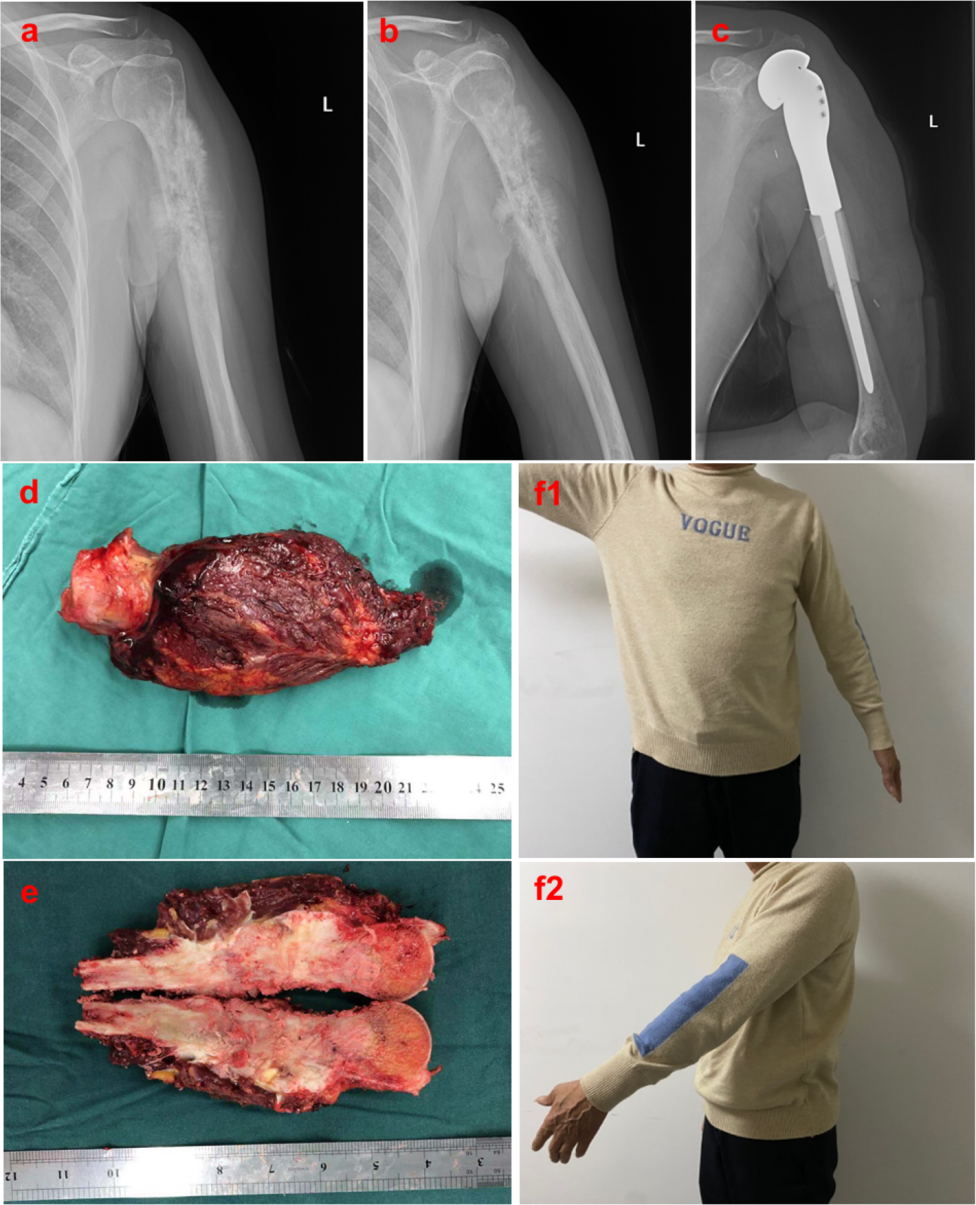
Case 1 (a, b) Preoperative AP X-ray indicates an osteogenic lesion and pathological fracture in the proximal humerus. (c) Six-month postoperative AP X-ray indicates stable joint function and alignment. (d, e) Surgical sample of the humeral osteosarcoma. (f1-2) Functional follow-up indicated stable joint function and alignment
